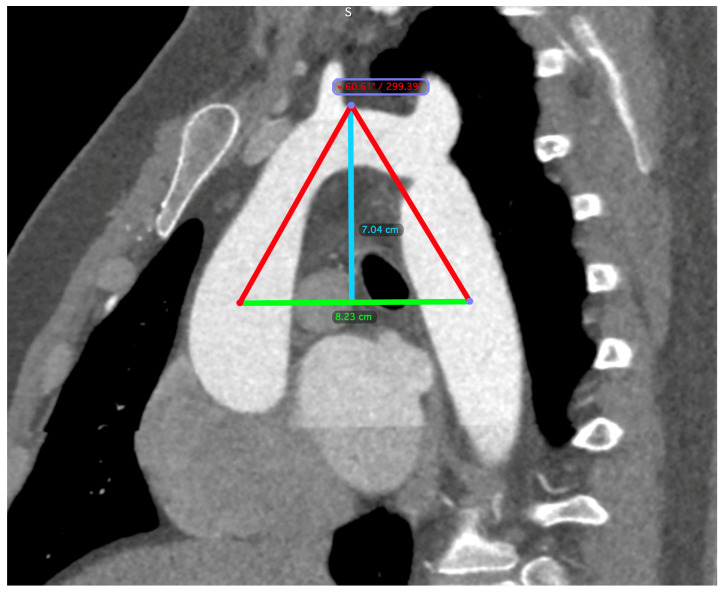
CT scan measurements. A triangle is formed between the apex of the arch (indicated by the blue line) and the midpoints of the ascending and descending aorta (indicated by the green line), which are aligned with the right pulmonary artery. The arch angle was defined as the vertex angle of the resultant triangle, and the arch width was the basal length of this triangle. The perpendicular distance from the basal length to the arch’s apex determined the arch height.
